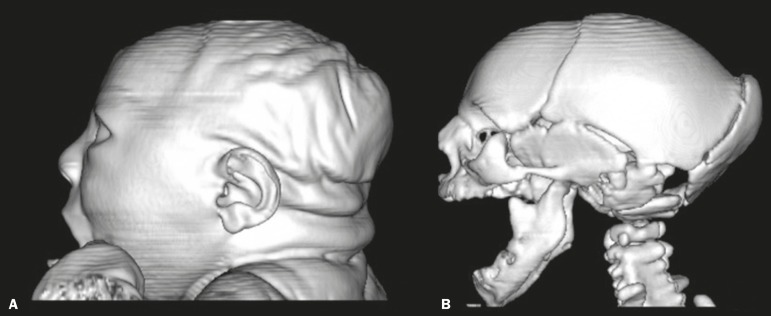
Three-dimensional reconstruction of head CT. A: Severe
